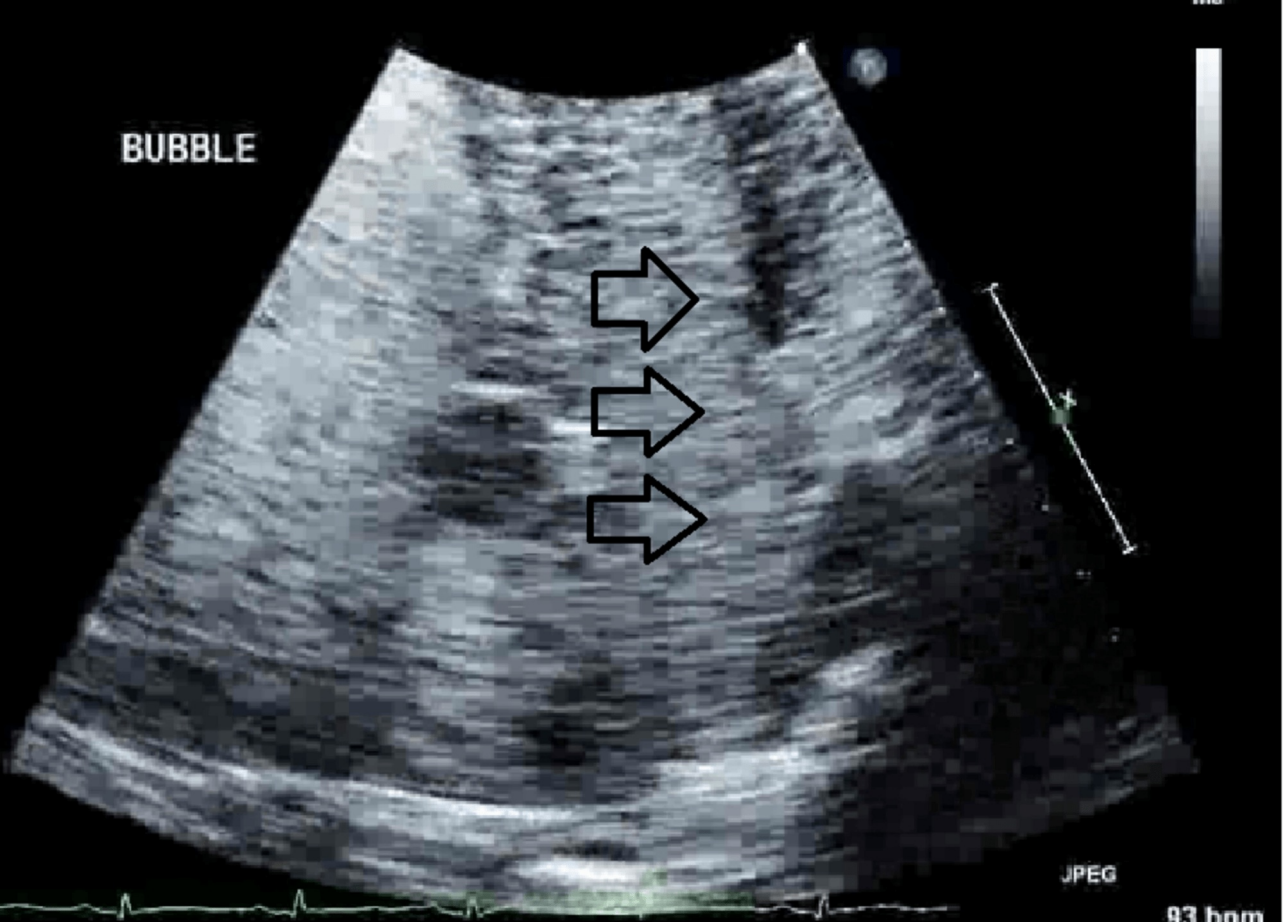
Transthoracic echocardiogram showing complete opacification of the left atrium and left ventricle within 2 cycles of injection of agitated saline contrast (arrows)
